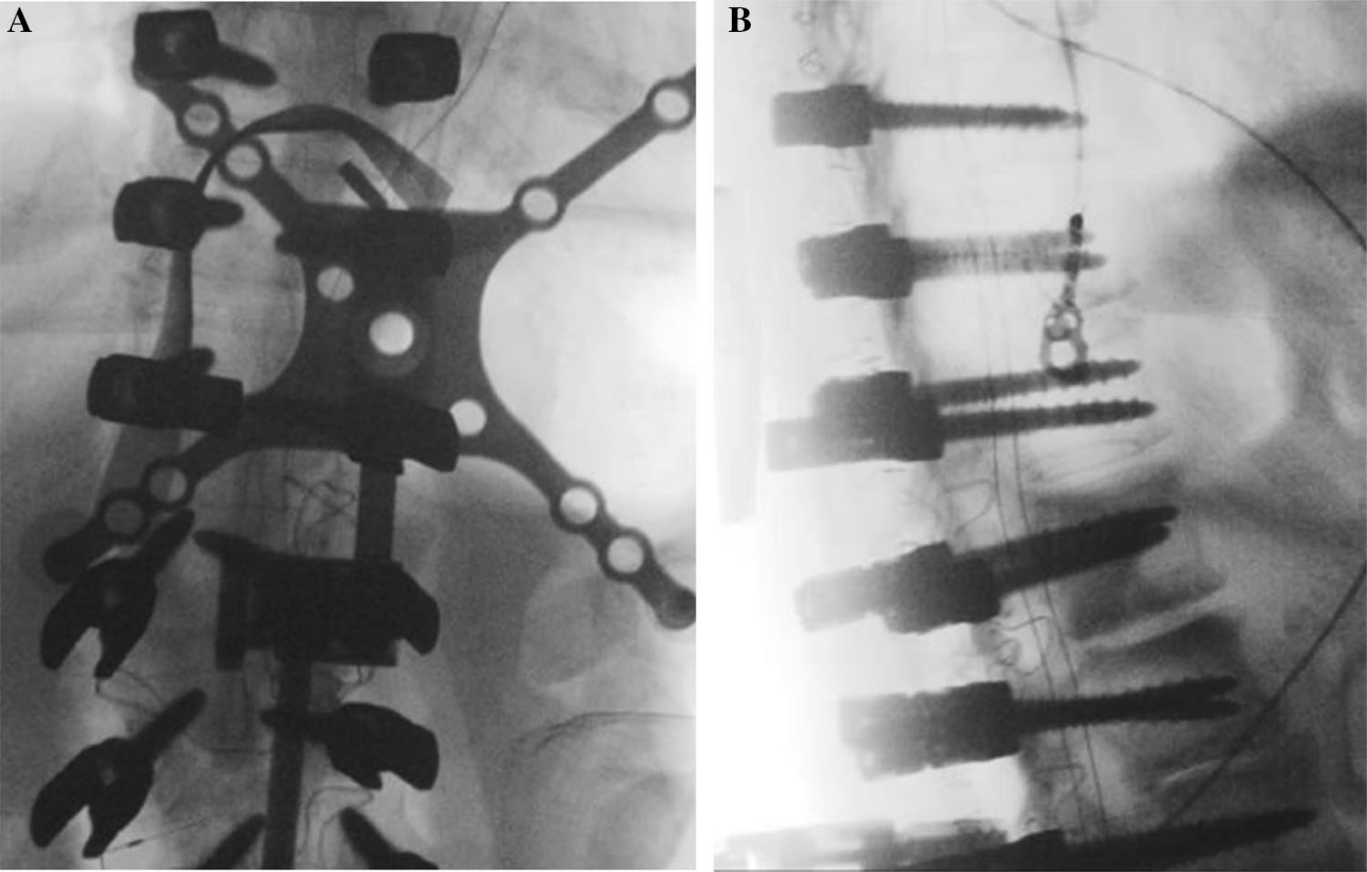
A, B Intraoperative AP and lateral scout image for CT. Spinous process array is also seen at the inferior of the image
